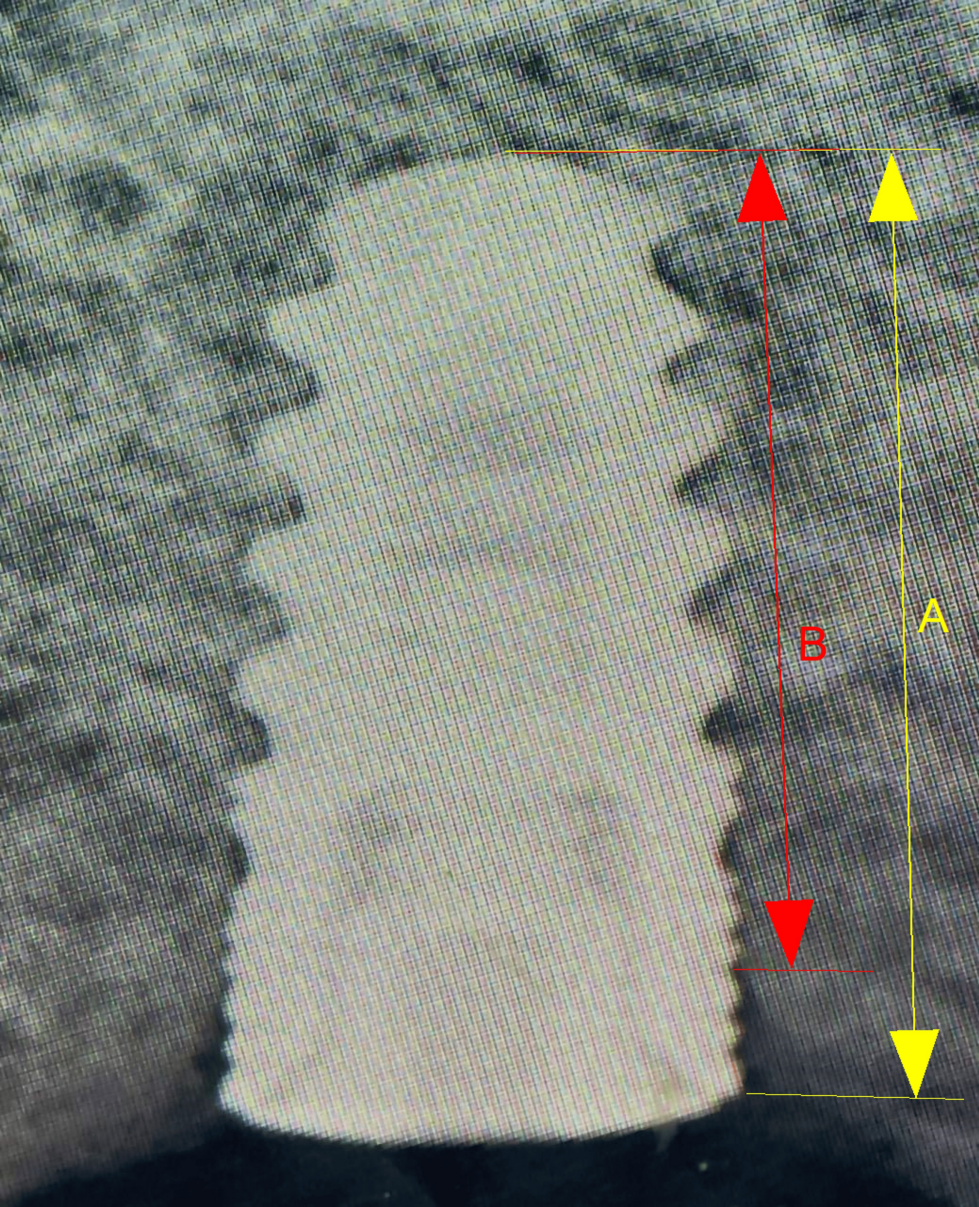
MBL assessment through dental radiograph A. Distance between the implant apex and the implant platform. B. Distance between the implant apex and first bone-to-implant contact. ImageJ software (National Institutes of Health, Bethesda, MD, USA) MBL, marginal bone loss
